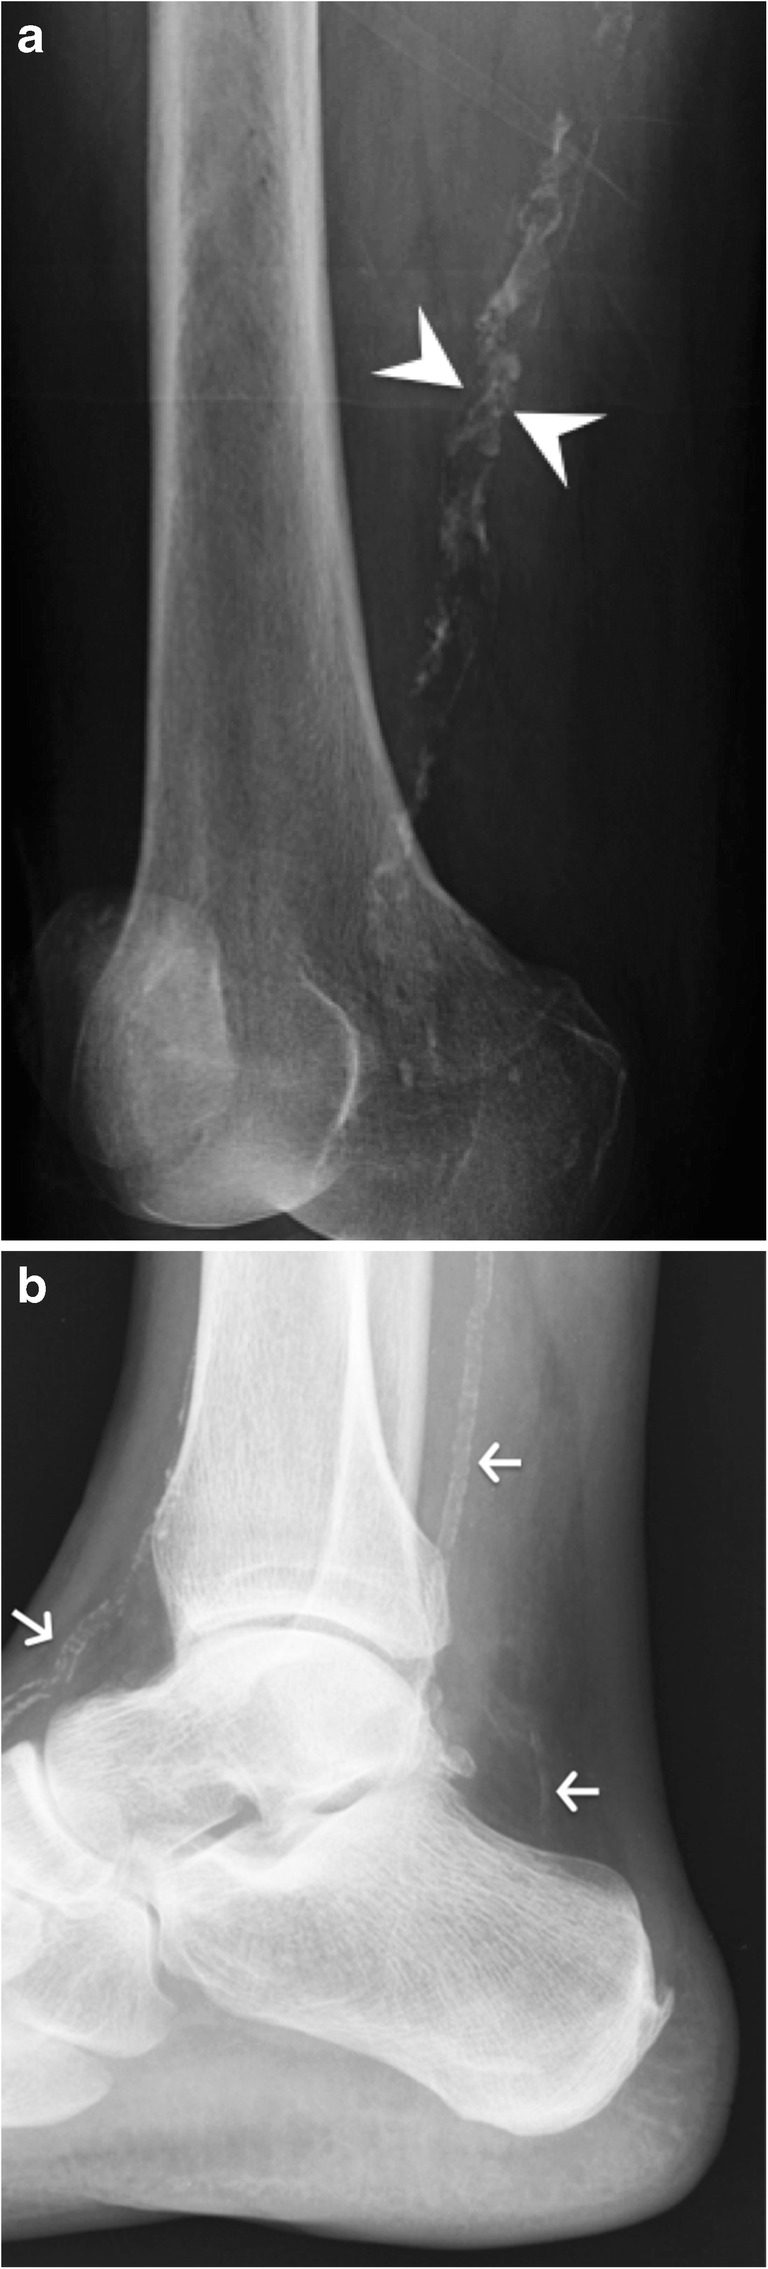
Vascular calcifications. (a) Oblique radiograph of the femur shows atherosclerotic vascular calcifications (arrowheads) and (b) lateral radiograph of the ankle shows metabolic vascular calcifications (arrows)
